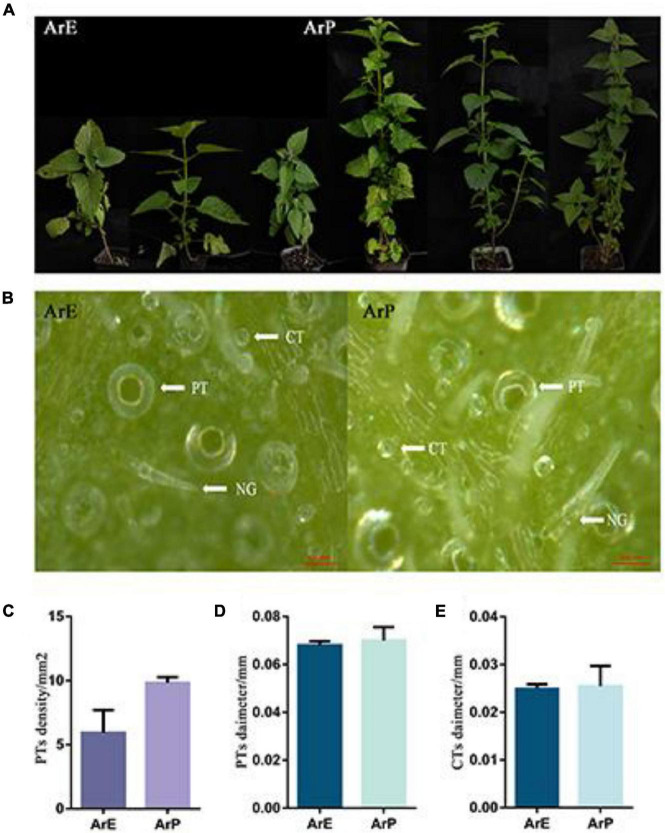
Morphological characterization of ArP and ArE of A. rugosa. (A) Photographs show three representative 12-week-old plants from the two chemotypes of *A. rugosa*. (B) Morphological features of trichomes on leaf surfaces, peltate trichome (PT), capitate trichome (CT), and non-glandular hairs (NG). (C) Peltate trichome density of ArE and ArP. Statistical significance was determined using the *t*-test (*P* < 0.05). (D) Peltate trichome diameter of ArE and ArP. Statistical significance was determined using the *t*-test (*P* > 0.05). (E) Capitate trichome diameter of ArE and ArP.
